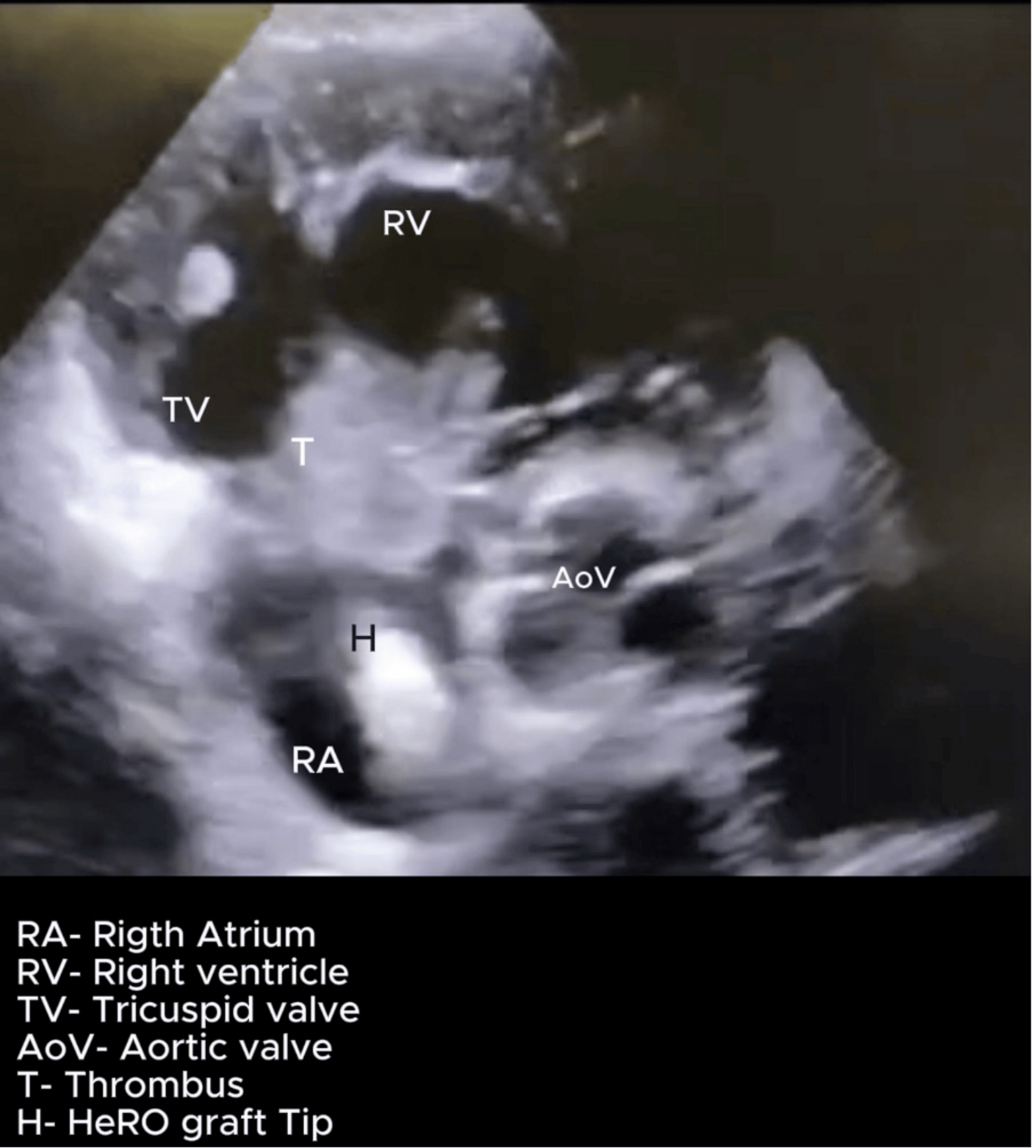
POCUS heart-parasternal short axis view at the aortic valve level showing HeRO graft tip and the thrombus attached to the tricuspid valve. Probe: Phased array. View: Parasternal short-axis view at the level of the aortic valve. Depth: 16 cm POCUS, point-of-care ultrasound; HeRO, Hemodialysis Reliable Outflow
